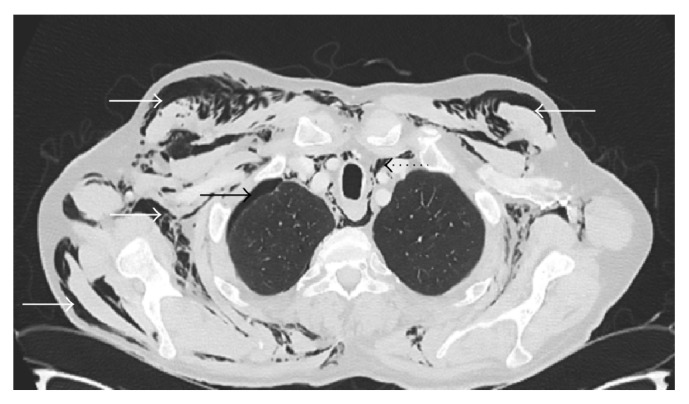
CT revealed diffuse subcutaneous emphysema (white arrows), pneumomediastinum (black dotted arrow), and a small pneumothorax of the right lung (black arrow).
